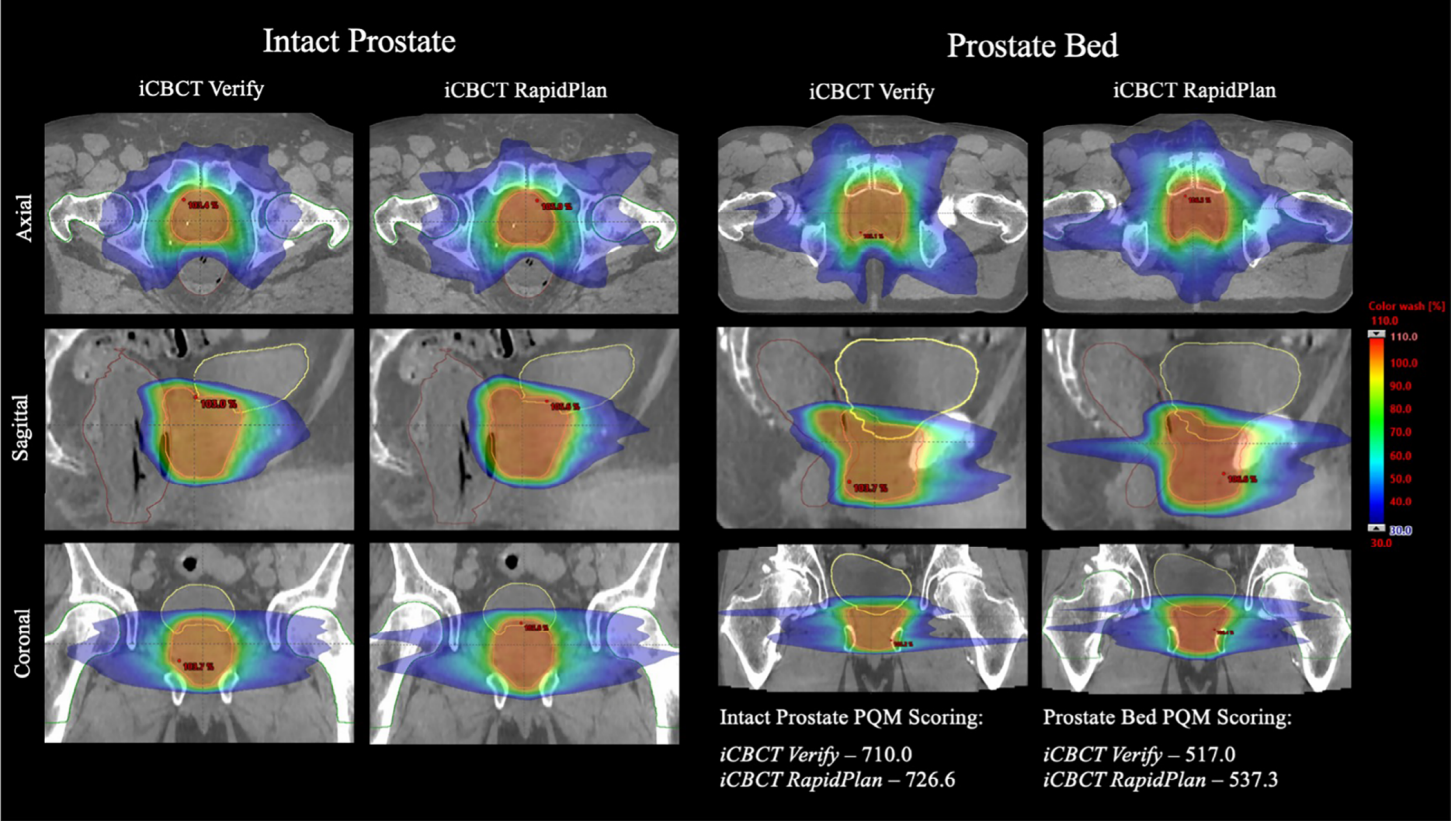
Comparison dose distributions with similar plan quality metric (PQM) scoring for each cohort of iCBCT Verify and iCBCT RapidPlan plans. Refined contours for relevant structure volumes are provided, including the planning target volume (pink), bladder (yellow), rectum (brown), and femoral heads (green). Color‐wash dose indicates the region of <110% and >30% of the prescribed dose.
